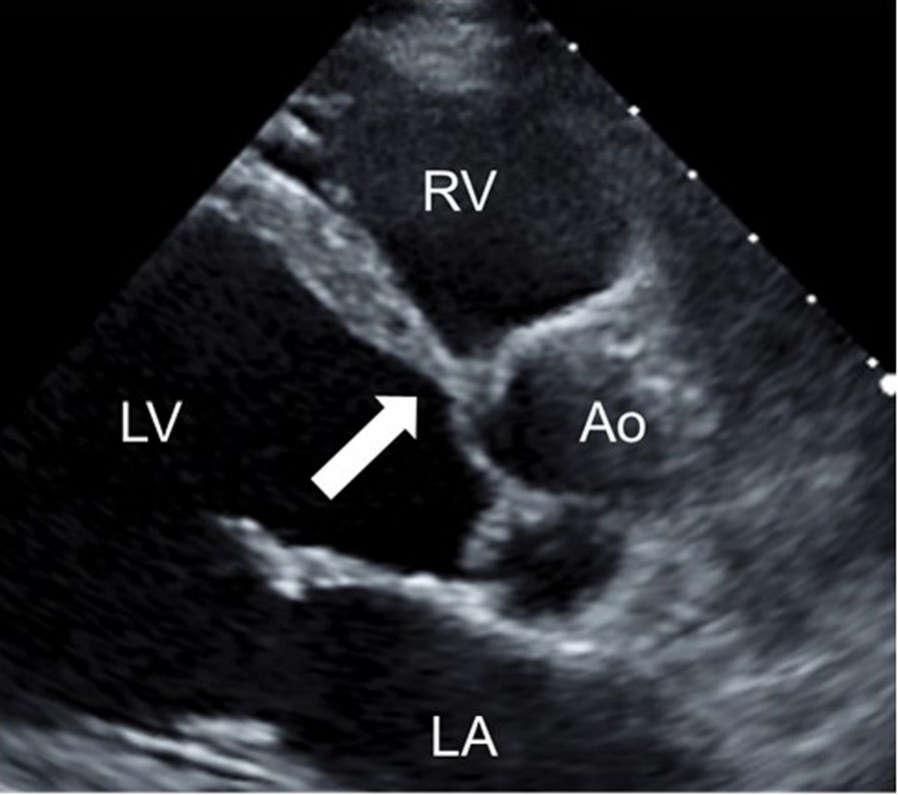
Abnormal thinning of the basal ventricular septum. A parasternal long-axis transthoracic echocardiography view at the level of the aortic valve showing abnormal ventricular septal thinning with a minimal thickness of 4.4 mm (arrow). LA left atrium, LV left ventricle, RA right atrium, RV right ventricle, Ao aorta
